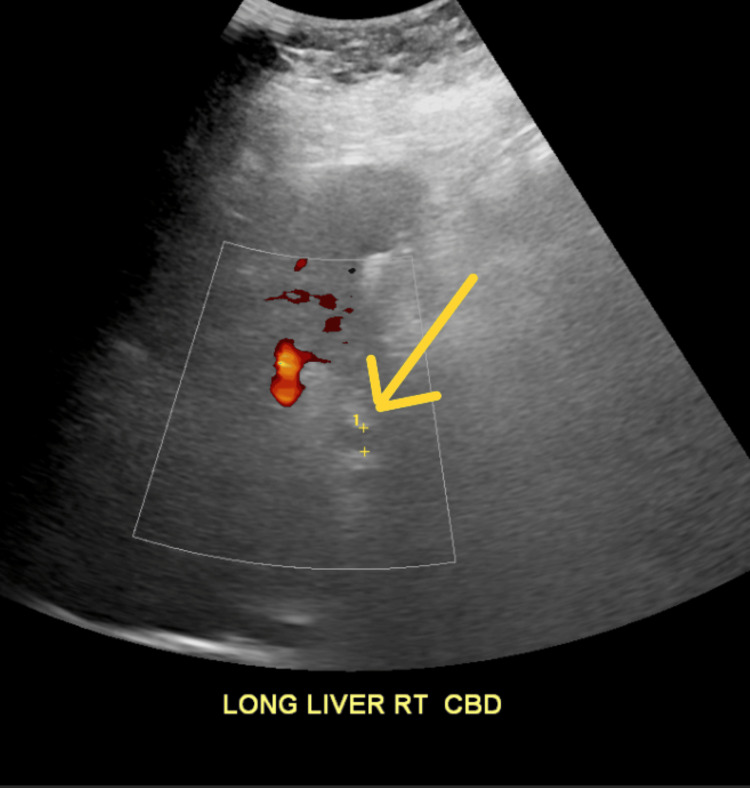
RUQ Abdominal Ultrasound of Liver Ultrasound of the abdomen showed increased echogenicity with nodular liver surface. The gallbladder is absent, consistent with prior cholecystectomy. The common bile duct measures 7 mm (yellow arrow). RUQ: right upper quadrant.
